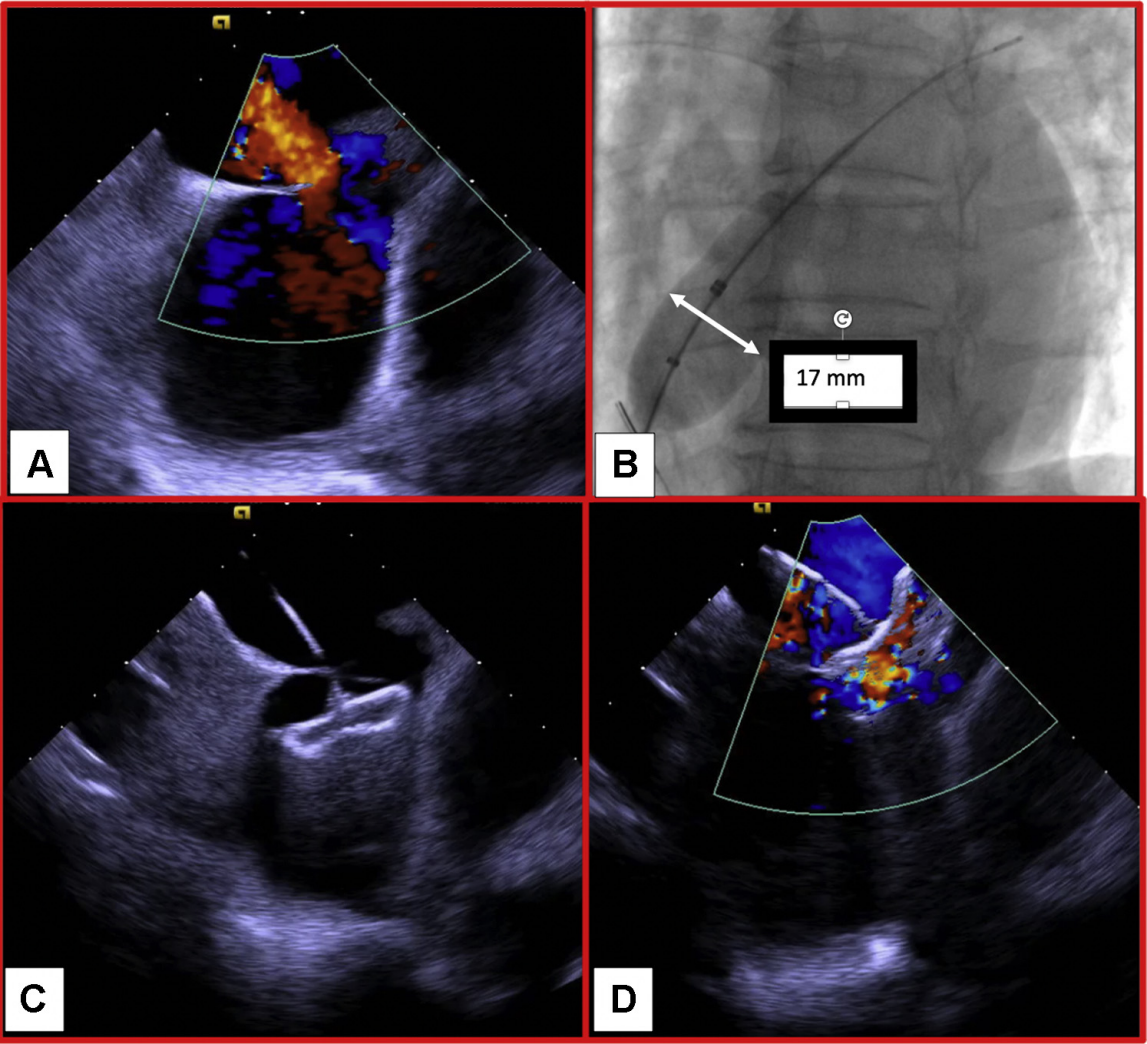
Intra-Operative Findings on Intracardiac Echocardiography (A) Intracardiac echocardiography showing left-to-right flow through malaligned atrial septal defect. (B) Fluoroscopic guided balloon-sizing of atrial septal defect. Intracardiac echocardiography images without (C) and with (D) color Doppler showing atrial septal occluder deployment.
